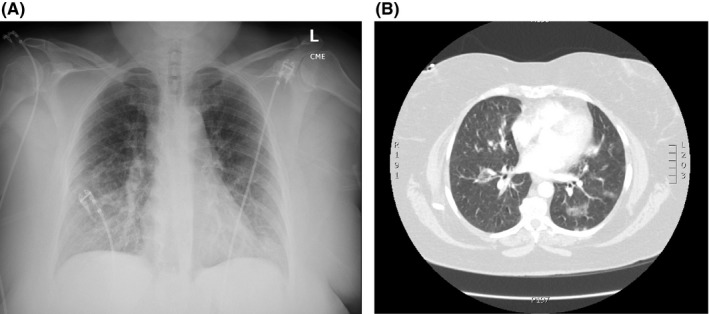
A, Portable chest X‐ray noting patchy interstitial and alveolar infiltrates. B, Chest CT‐Angiogram (Pulmonary embolism protocol) with multiple peripherally based groundglass multilobar opacities
